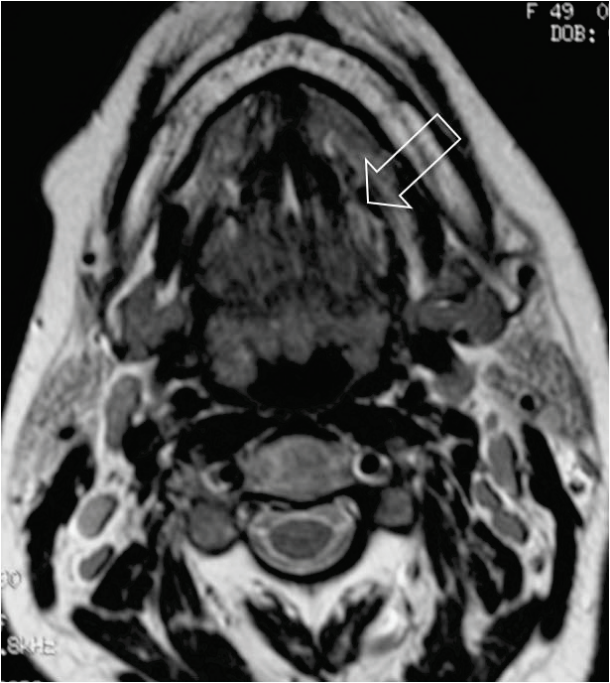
Tongue magnetic resonance image showing the neoformation (arrow).
